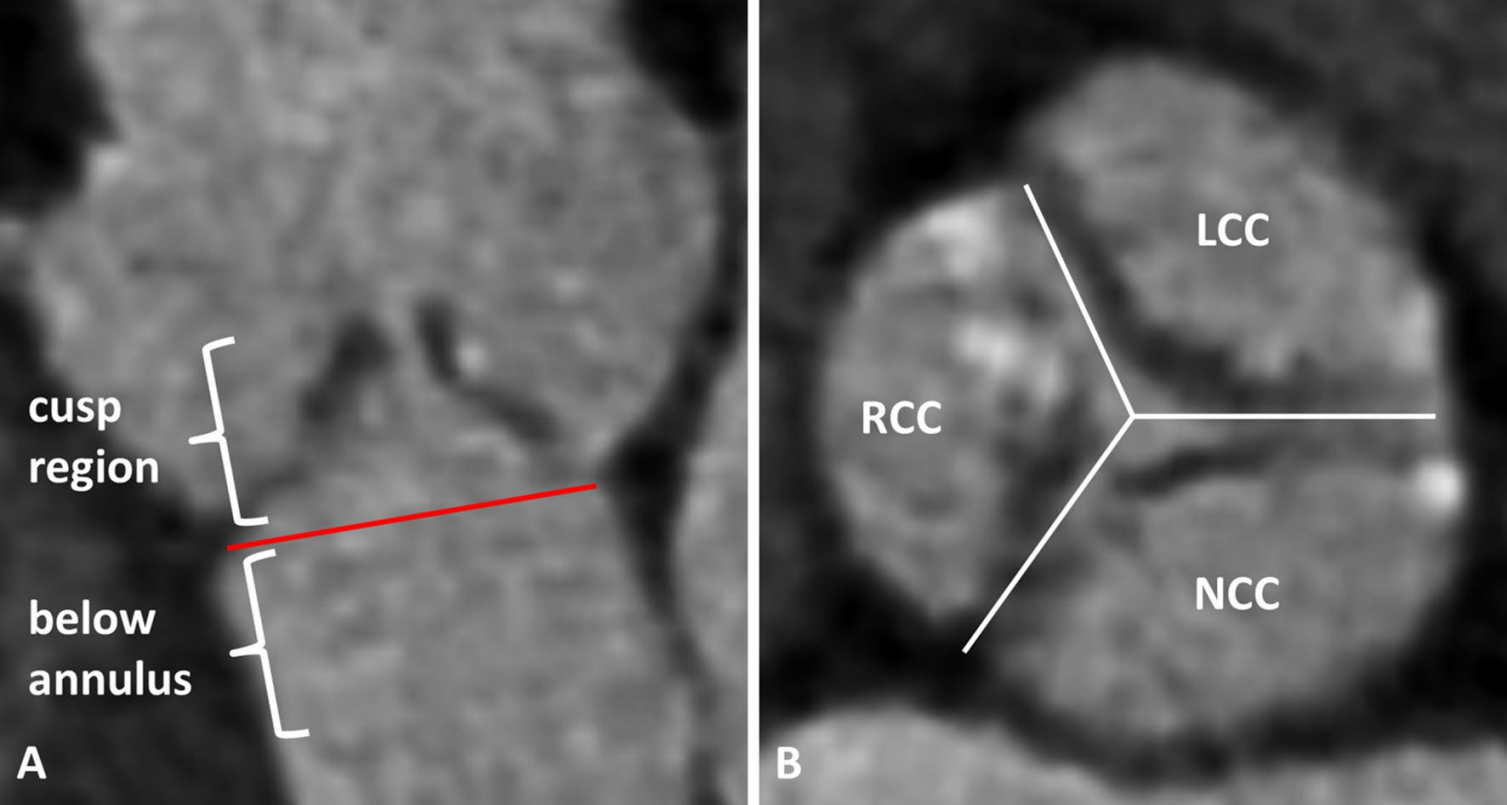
Calcification assessment of the device landing zone. Pre-TAVI contrast-enhanced CTA sagittal oblique and axial reconstruction delineating the two different heights of the device landing zone (a red line delineating the annulus plane) and the division of the aortic valve into three cups for the semiquantitative calcification scoring (b)
